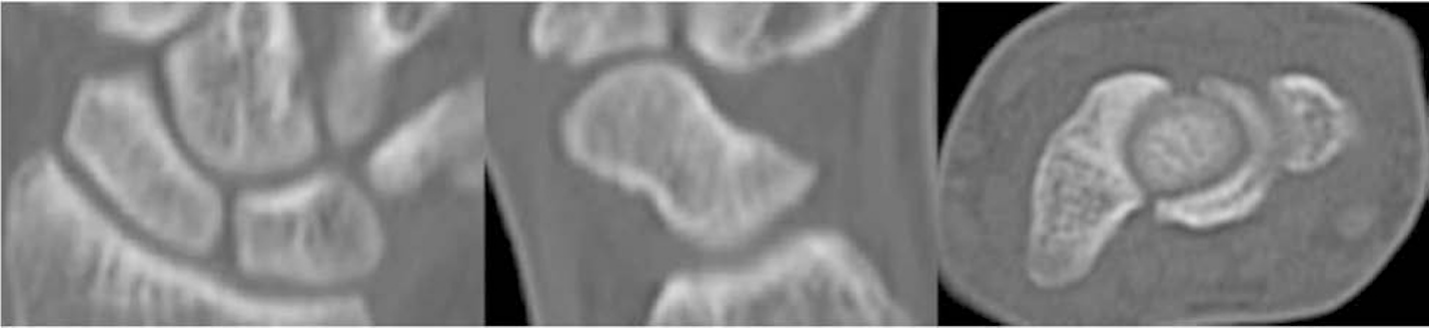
Computed tomography performed 3 months after the initial presentation. The image shows complete healing of the fracture
